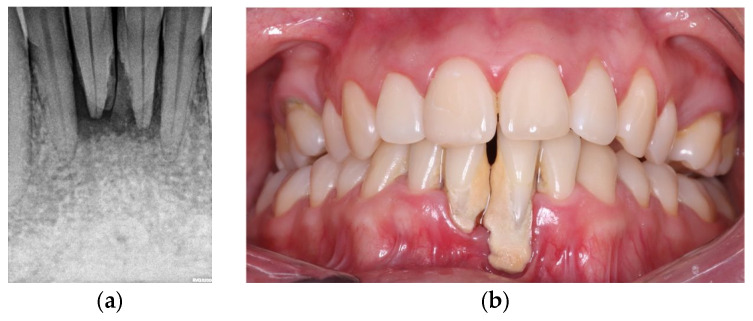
(a) Periapical radiograph of lower incisors (3.2–4.1) showing periapical process and one-wall intrabony defect. (b) Localized clinical attachment loss associated with labial piercing.
